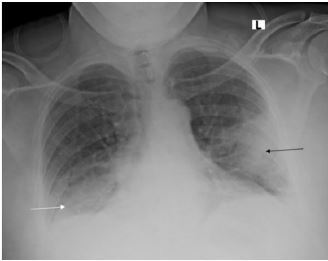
Chest radiograph with bilateral infiltrates involving the left mid to lower lung (black arrow) and the right lung base (white arrow), suggestive of multifocal or viral pneumonia.
